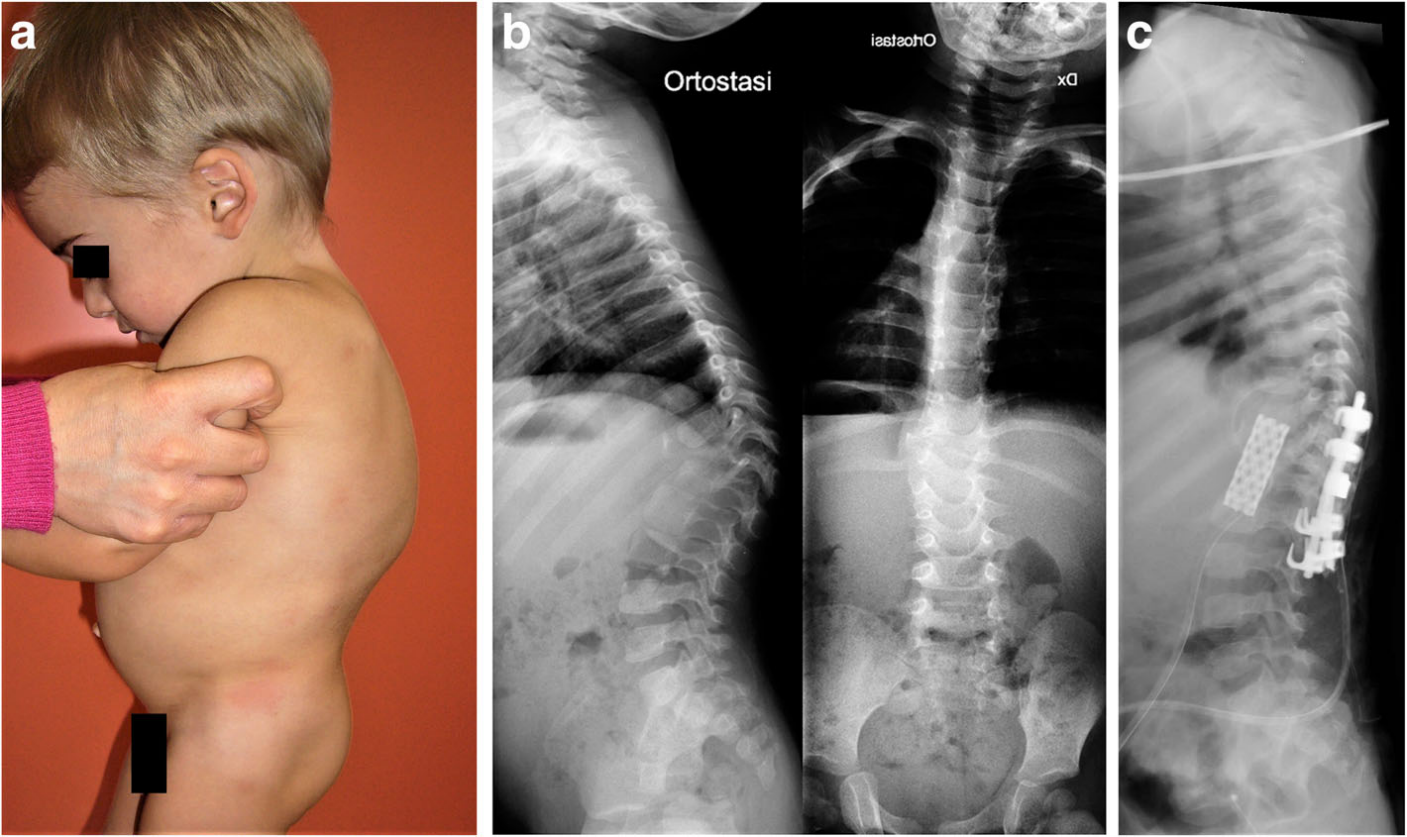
Pre-operatory clinical aspect (a), plain radiographs at 2 years of age showing the persistent and progressive kyphosis (b) and post-operatory standing lateral radiograph showing the double approach with posterior hooks and anterior bank bone graft titanium cage from T11 to L2 (c)
